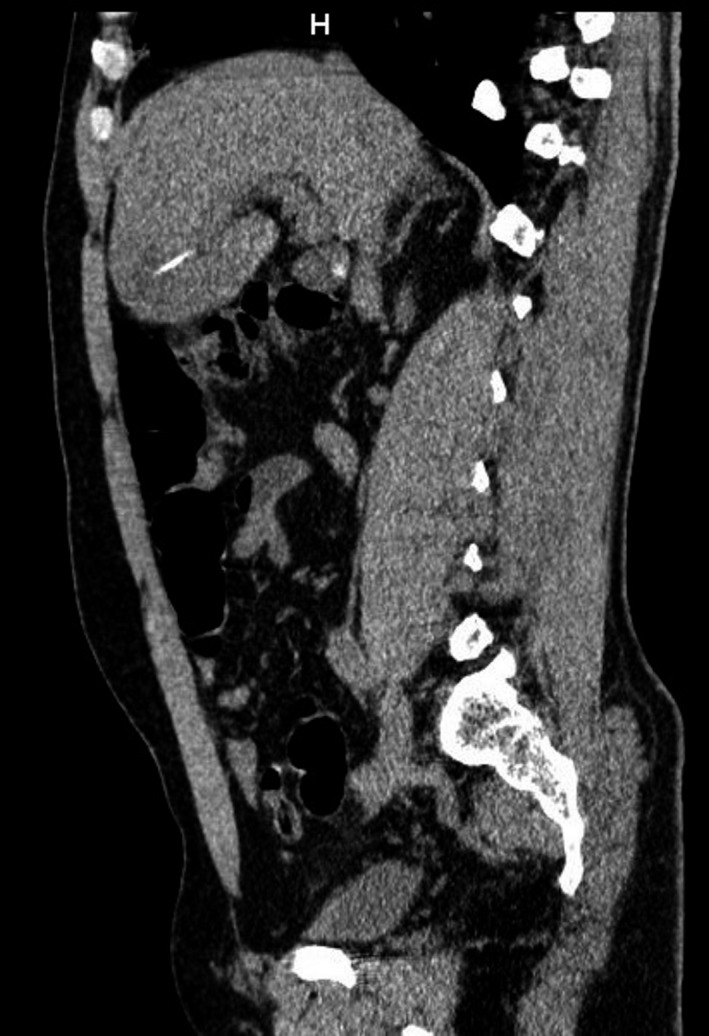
Computed tomography sagital image showing a linear calcified body inside the liver abscess
